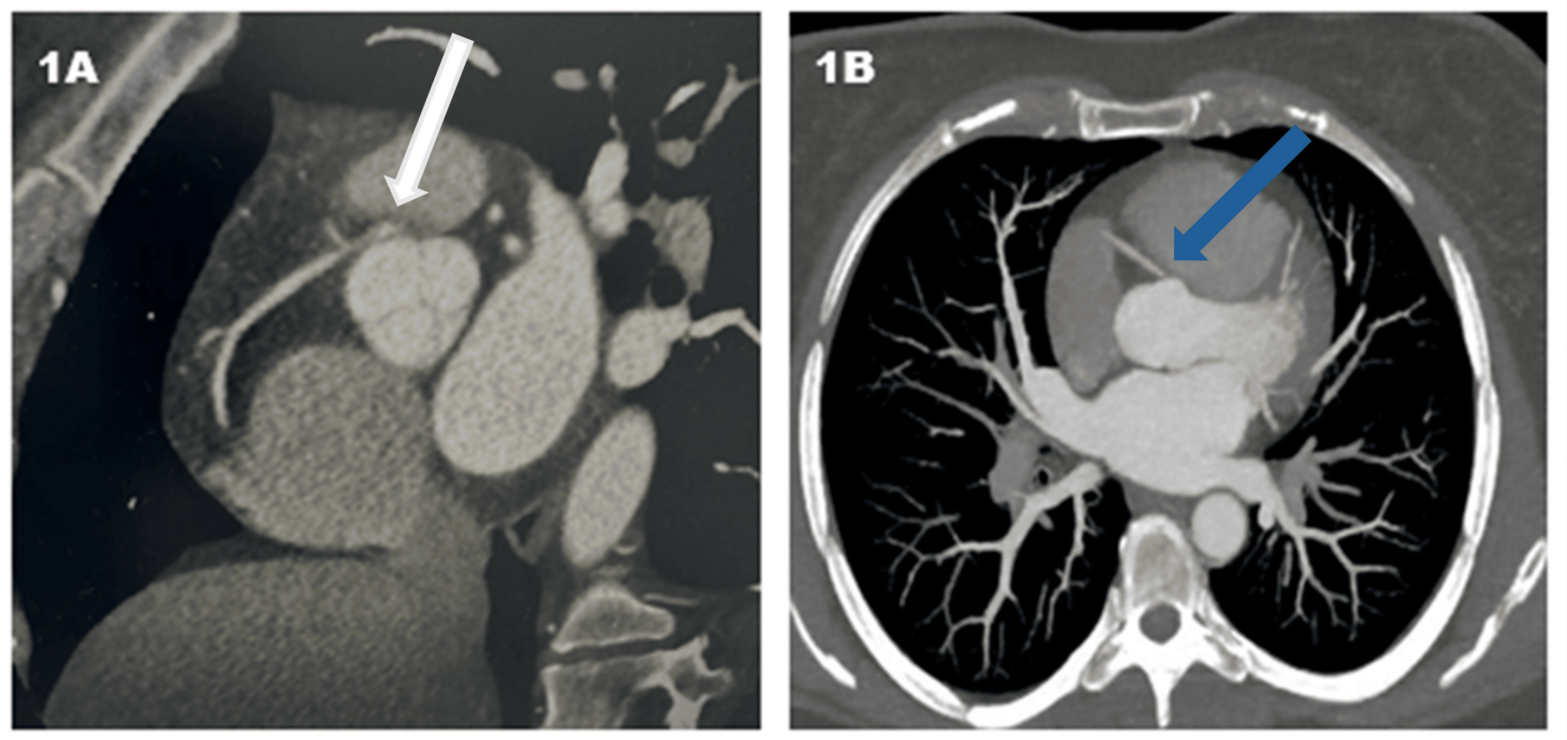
Case 1 Imaging (A) Coronary computed tomography angiography (CTA) showing right coronary artery originating from the left coronary cusp with an anterior course with compression between the aorta and right ventricular outflow tract (white arrow). (B) Chest CTA showing anterior course of right coronary artery (RCA) originating from the left coronary cusp (blue arrow).
